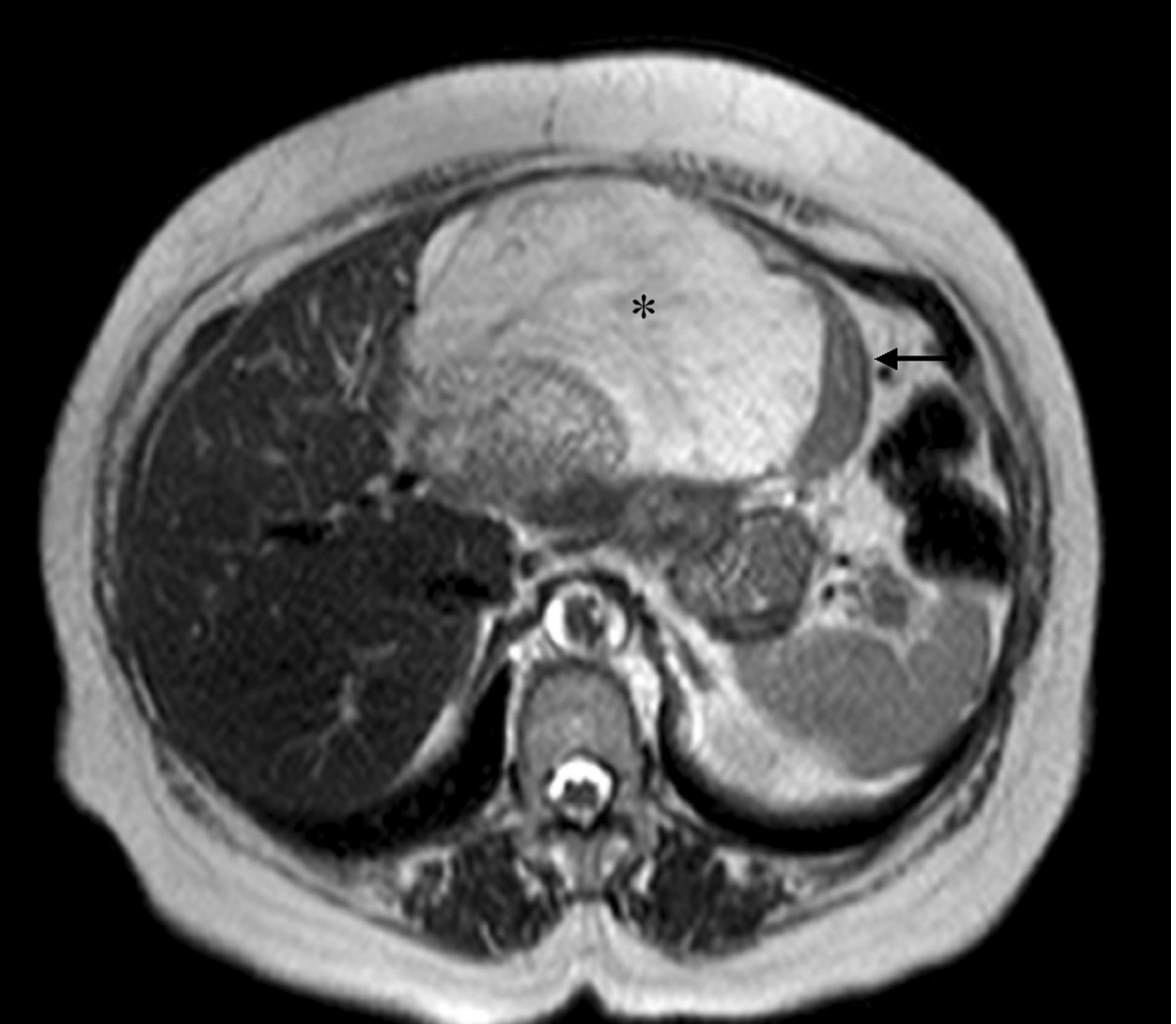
MR imaging sequence with T2-weighted transversal. Imaging shows a hypointense signal originating in the peripheral region (arrow) and a dominant component associated with a hyperintense signal (*).
